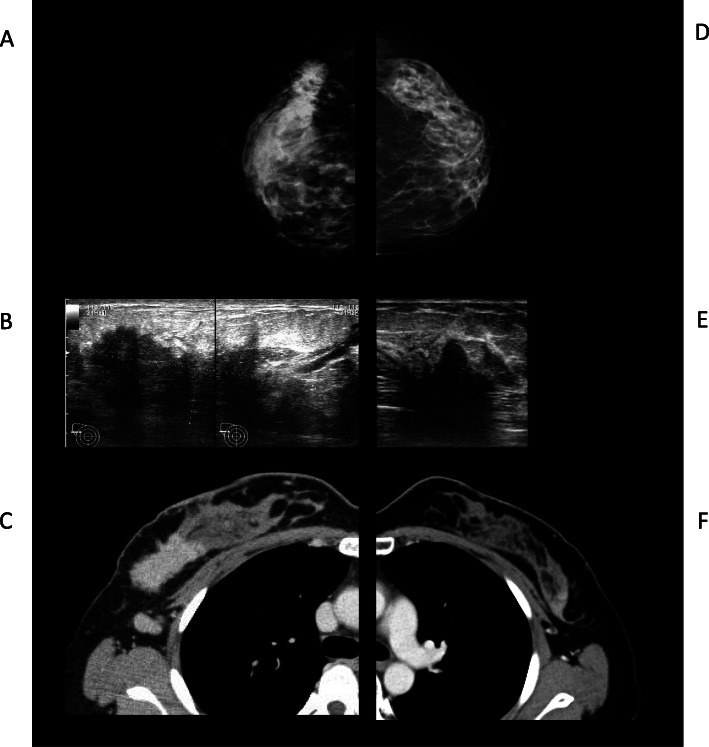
Preoperative imaging of right and left breast lesions. Mammography (cranio-caudal view) revealed a mass in the middle outer portion of the right breast (a). No lesions were detected in the left breast on mammography (d). Ultrasonography showed a 59-mm hypoechoic mass with an unclear margin in the right breast (b), and an 18-mm hypoechoic lesion with an unclear margin in the left breast (e). Computed tomography (CT) with contrast revealed a 53-mm mass in the right breast (c). Several axillary lymph node metastases were detected, but no other metastasis, on CT. In the left breast, CT with contrast demonstrated a 34-mm mass but no other metastatic lesions (f)
